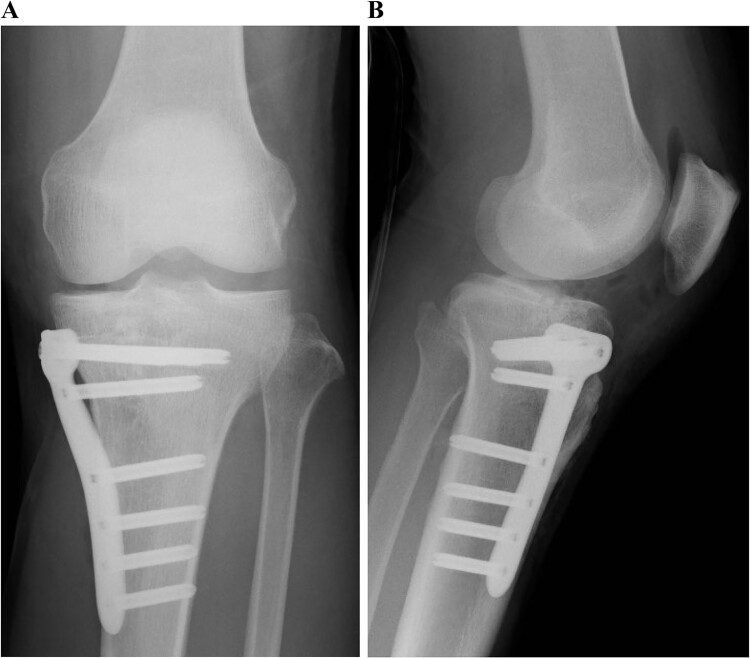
Postoperative plain radiographs of the left knee. Internal fixation was performed with the TriS Medial HTO Plate System.
